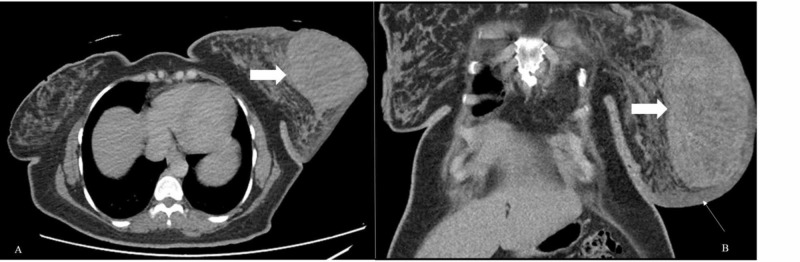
Contrast-enhanced CT scan Axial (A) and coronal (B) images show large enhancing soft tissue mass in the left breast (thick white arrows). Marked skin thickening is also noted (long white arrow) as well as left breast edema.
